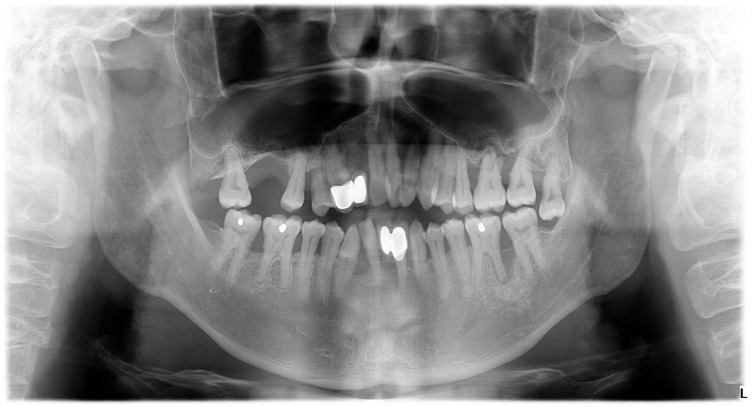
Case 2—Baseline radiographs. OPG taken in August 2020 at primary care.
